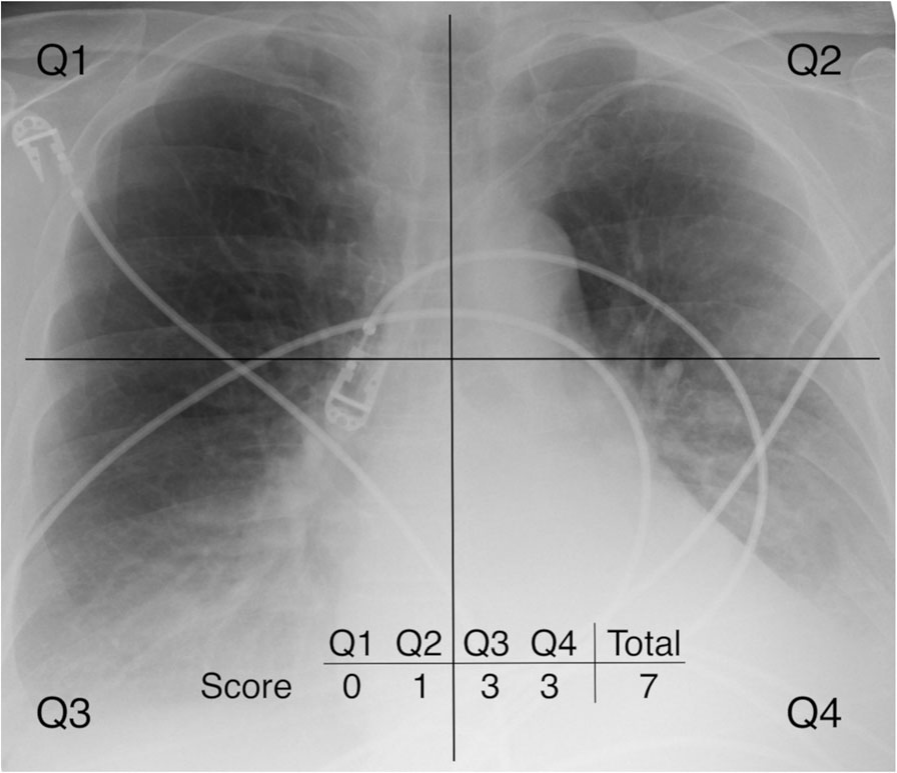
An example of a scored chest radiograph. Each image is divided into four quadrants (Q) with each quadrant assigned a score from 0 to 4. The total score, representing the sum of the four quadrants, was used as a predictor in our clinical outcomes models
